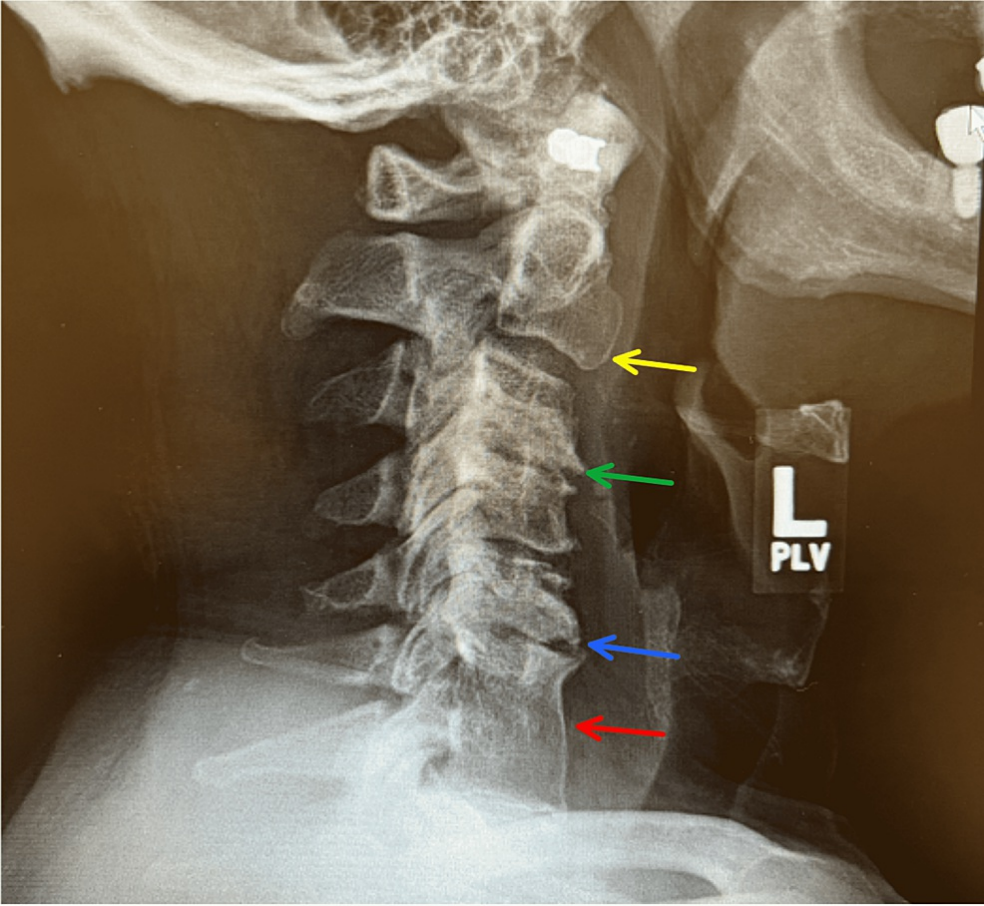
X-ray of the cervical spine Grade 1 C2 on C3 anterolisthesis (yellow arrow). Severe multilevel degenerative changes, particularly C3-C4 (green arrow) and C5-C6 (blue arrow). Narrowing of the disc spaces, anterior dorsal endplate with sclerotic degenerative changes. The old C6-C7 discectomy and fusion (red arrow).
